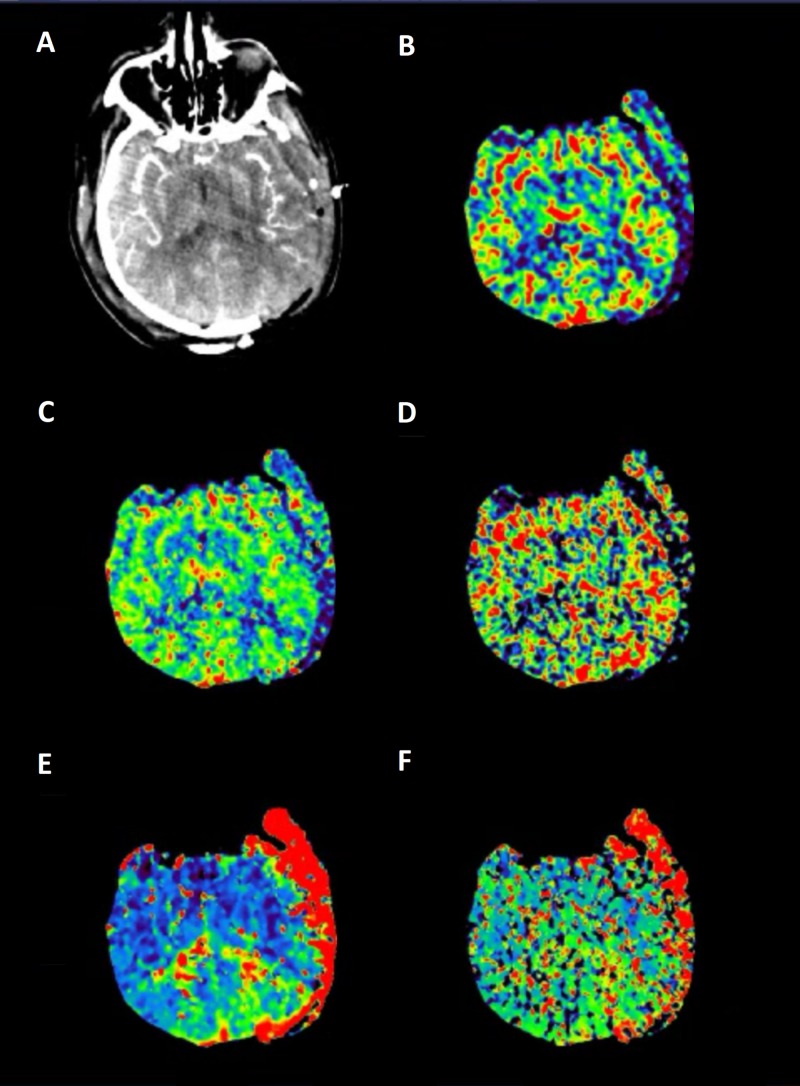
Computed tomography perfusion demonstrating (from left to right) (A) CTA, (B) CBV, (C) CBF, (D) MTT, (E) TTP, and (F) delay CBF: cerebral blood flow; CBV: cerebral blood volume; CTA: computed tomography angiogram; MTT: mean transit time; TTP: time to peak
